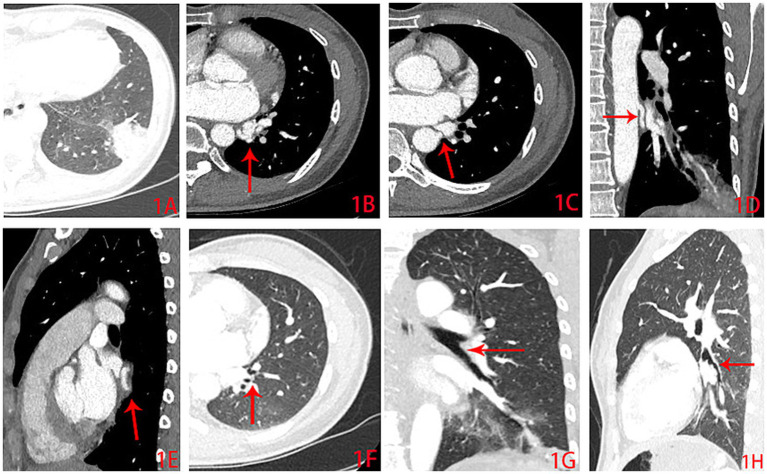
CT angiography images of the thoracic aorta (CTA). (A) The lung window of the CTA shows localized increase in density in the lower lateral segment of the left lung, with most areas showing consolidation and a small amount of ground-glass opacity; (B,C) The mediastinal window shows a dilated and tortuous vessel originating from the descending aorta; The arrows in (C) indicate the starting point of the abnormal arterial branch; (D) The coronal imaging shows the abnormal artery originating from the thoracic aorta and proceeding downward to participate in the blood supply of the left lower lung; (E) The abnormal artery partly shown in the sagittal image; (F–H) The bronchial tree structure of the lower lateral segment of the left lung was normal.
